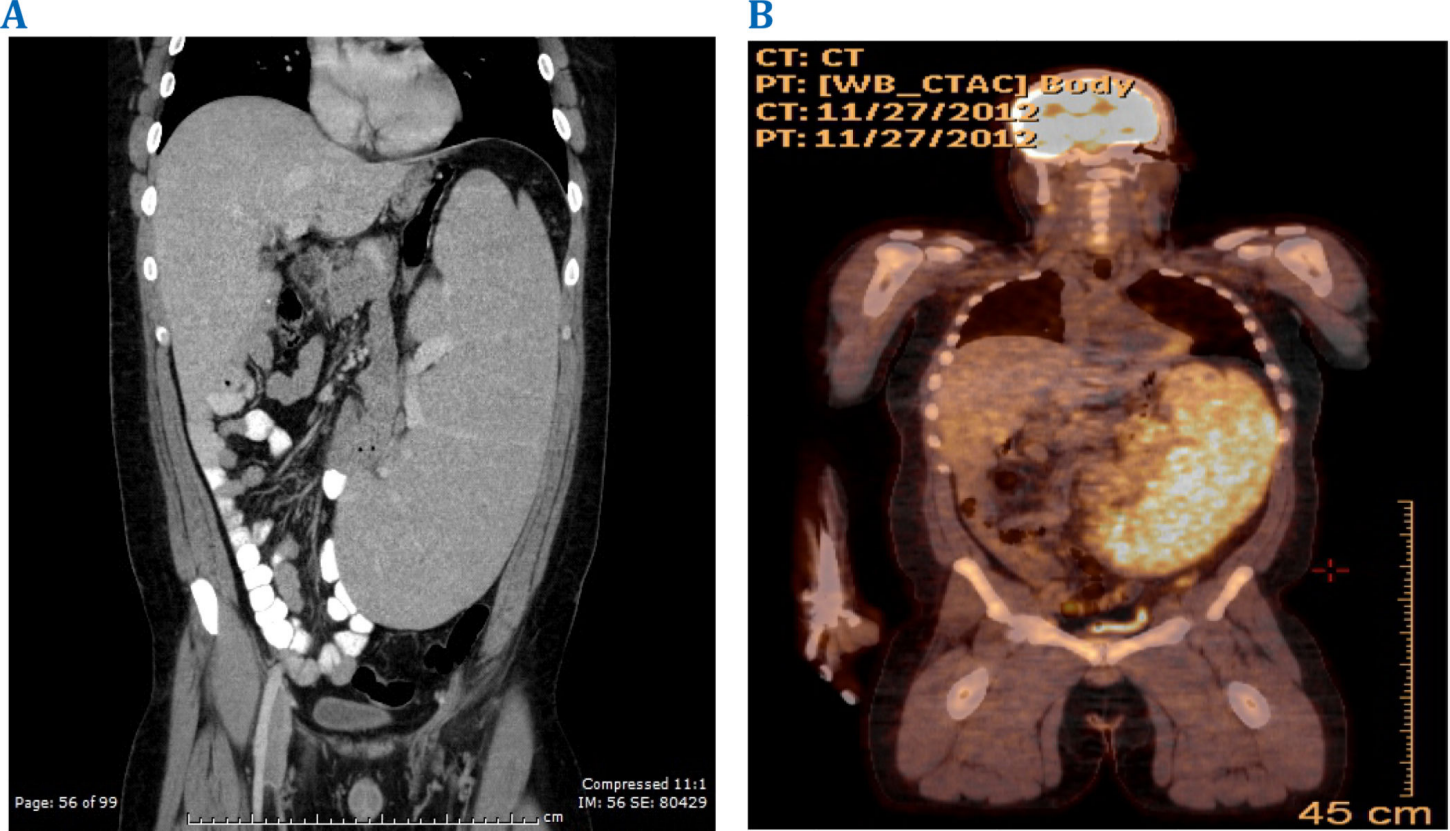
CT and PET imaging reveal massive splenomegaly.
